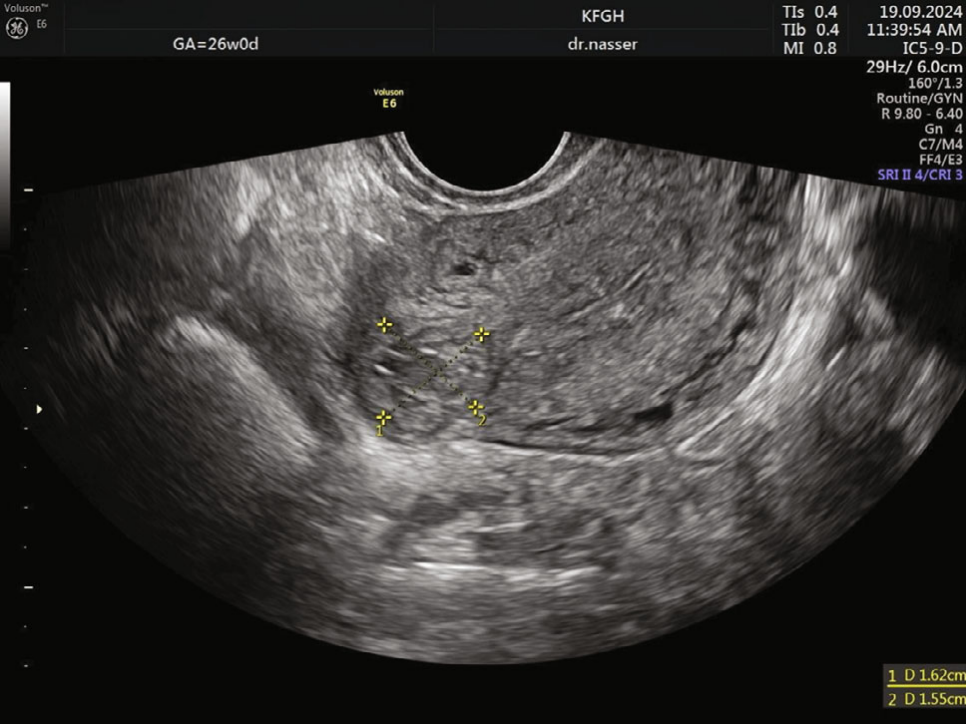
Ultrasound image of an interstitial ectopic pregnancy 3 months after the initiation of methotrexate therapy.
